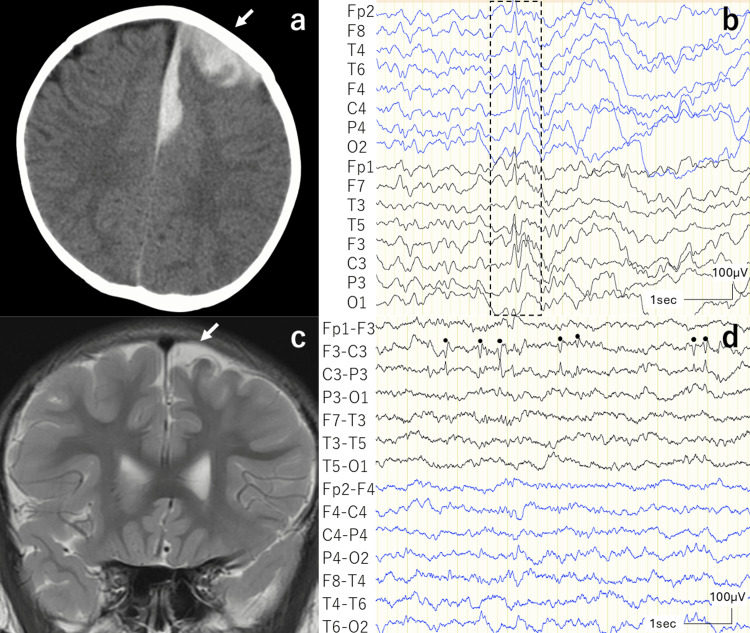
CT, MRI, and EEG findings of Case 1 (a) CT scan at the first convulsion showed subdural hematomas (SDH) in the left frontal lobe (arrow). (b) Referential montage EEG conducted one and a half years after discontinuation of sodium valproate (VPA) showed bilateral frontal spikes (surrounded by the dotted line). (c) Mild atrophy in the left frontal lobe was seen in follow-up MRI (arrow). (d) Bipolar montage EEG at follow-up showed left central spikes (dot).
